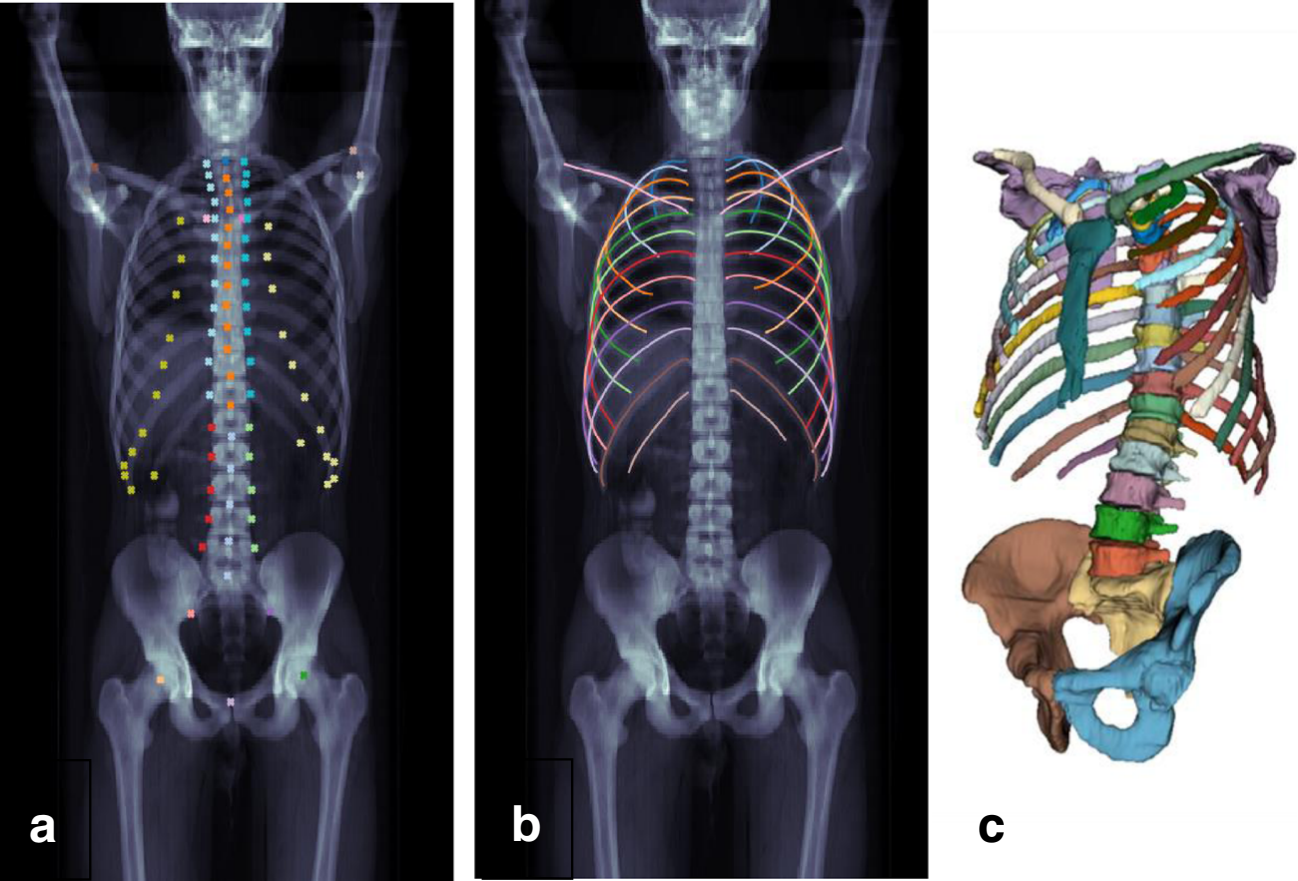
a Maximum-intensity projection of a CT scan with annotated landmarks. b Detected center lines for ribs and clavicles. c Surface reconstruction of the resulting segmentation enabling calculation of the skeletal volume in relation to which the sum of the metabolically active volume of bone lesions is expressed. Reproduced with permission [15]
